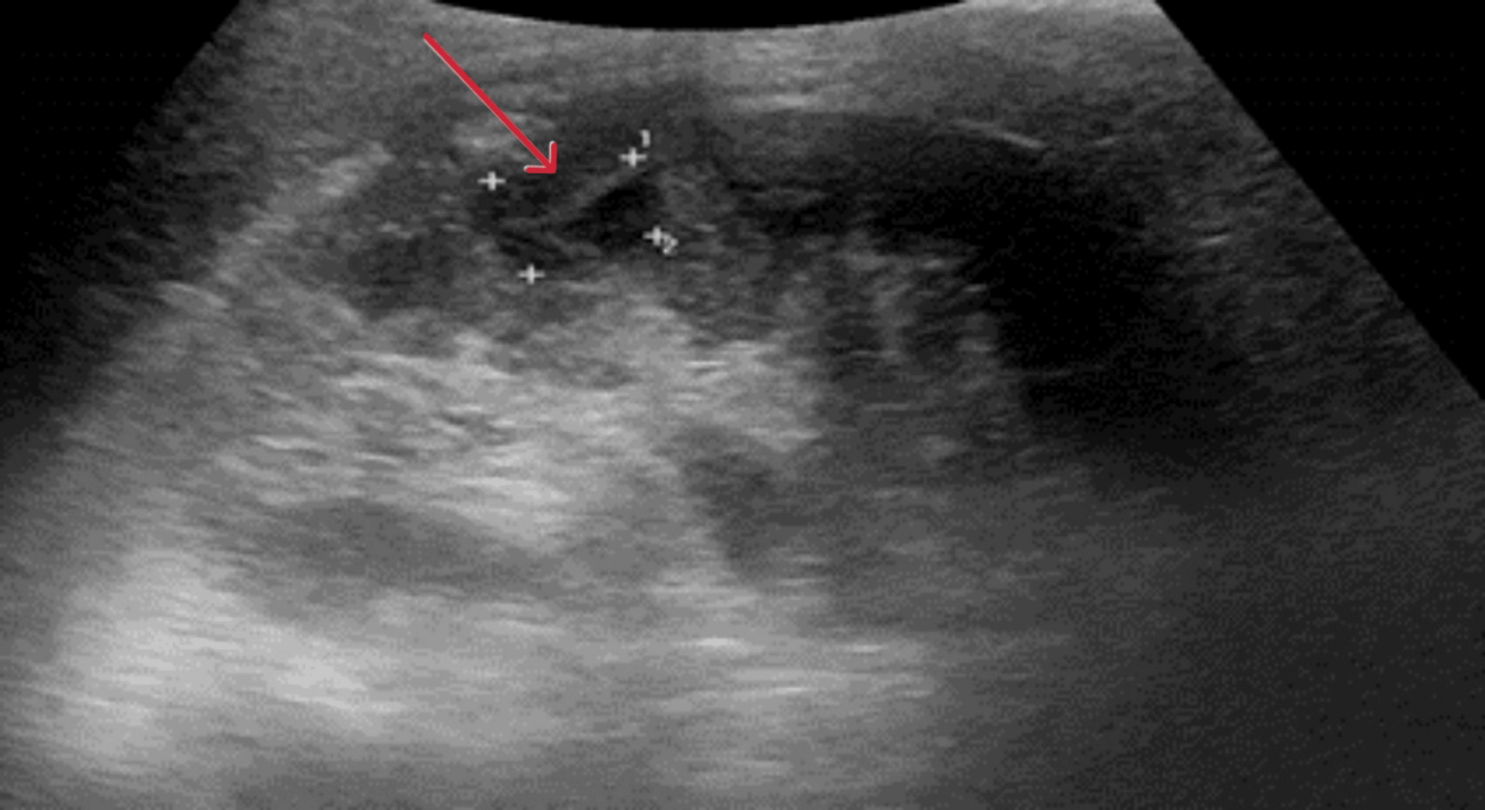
US KUB Day 46 showing the left renal abscess reduced in size, measuring 20 x 16 x 14 mm in size (tr x p x depth). Red arrow showing the presence of a left renal abscess. US: ultrasound; KUB: kidney, ureter, and bladder
